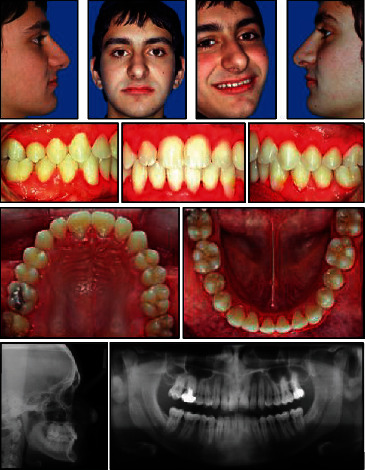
Posttreatment facial and intraoral photographs and X-rays.
